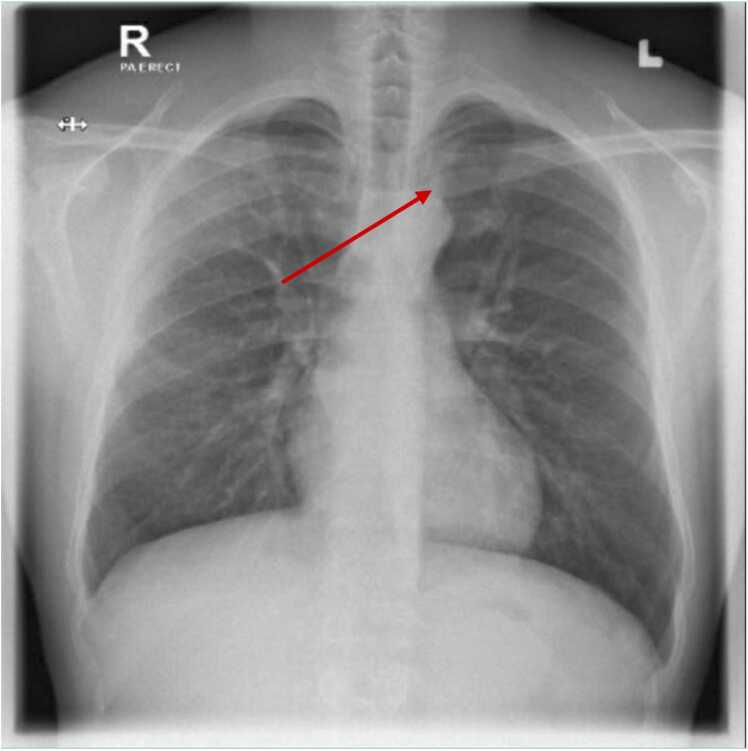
Chest X-ray demonstrating hazy opacities and inhomogeneous infiltrations in the right upper lung.
